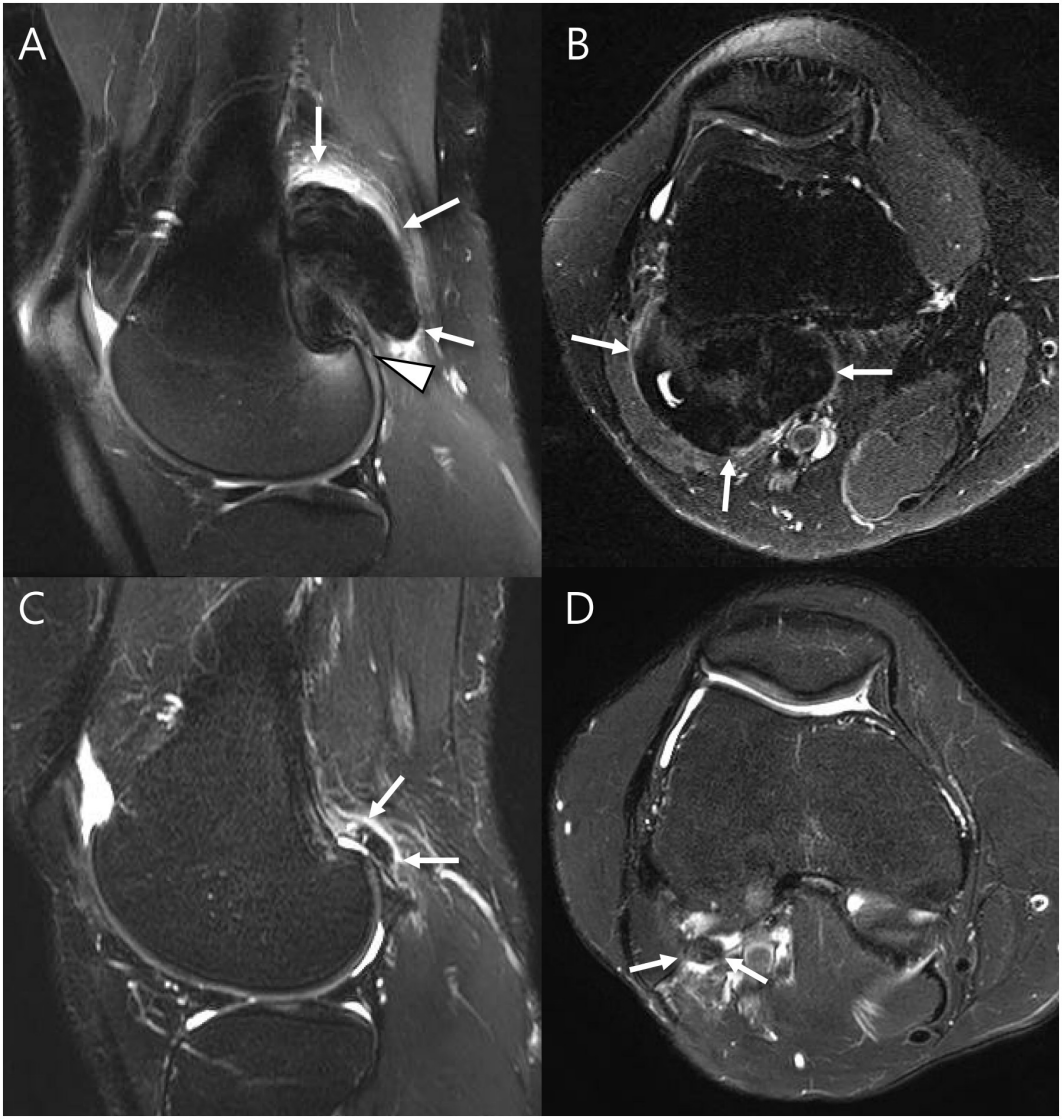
A 29-year old woman with pathology-proven extraarticular D-TSGCT of knee. (A and B) Preoperative sagittal and axial T2-weighted images with fat suppression show soft tissue mass that are very low signal intensity located in the extraarticular posterolateral aspect of knee with infiltrative margin containing granular internal hypointensity without visible peripheral hypointensity (arrows). Note that the lateral femoral condyle cartilage is involved by tumor (arrowhead). (C and D) Follow-up MRI after 30 months show recurrent mass with same nature (arrows).
